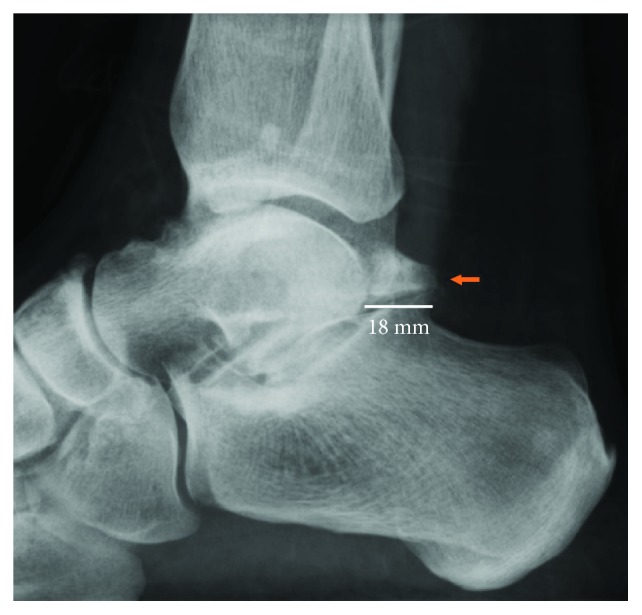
X-ray of the hind foot, lateral view, showing a 1.8 cm accessory posterior bone fragment (arrow).
